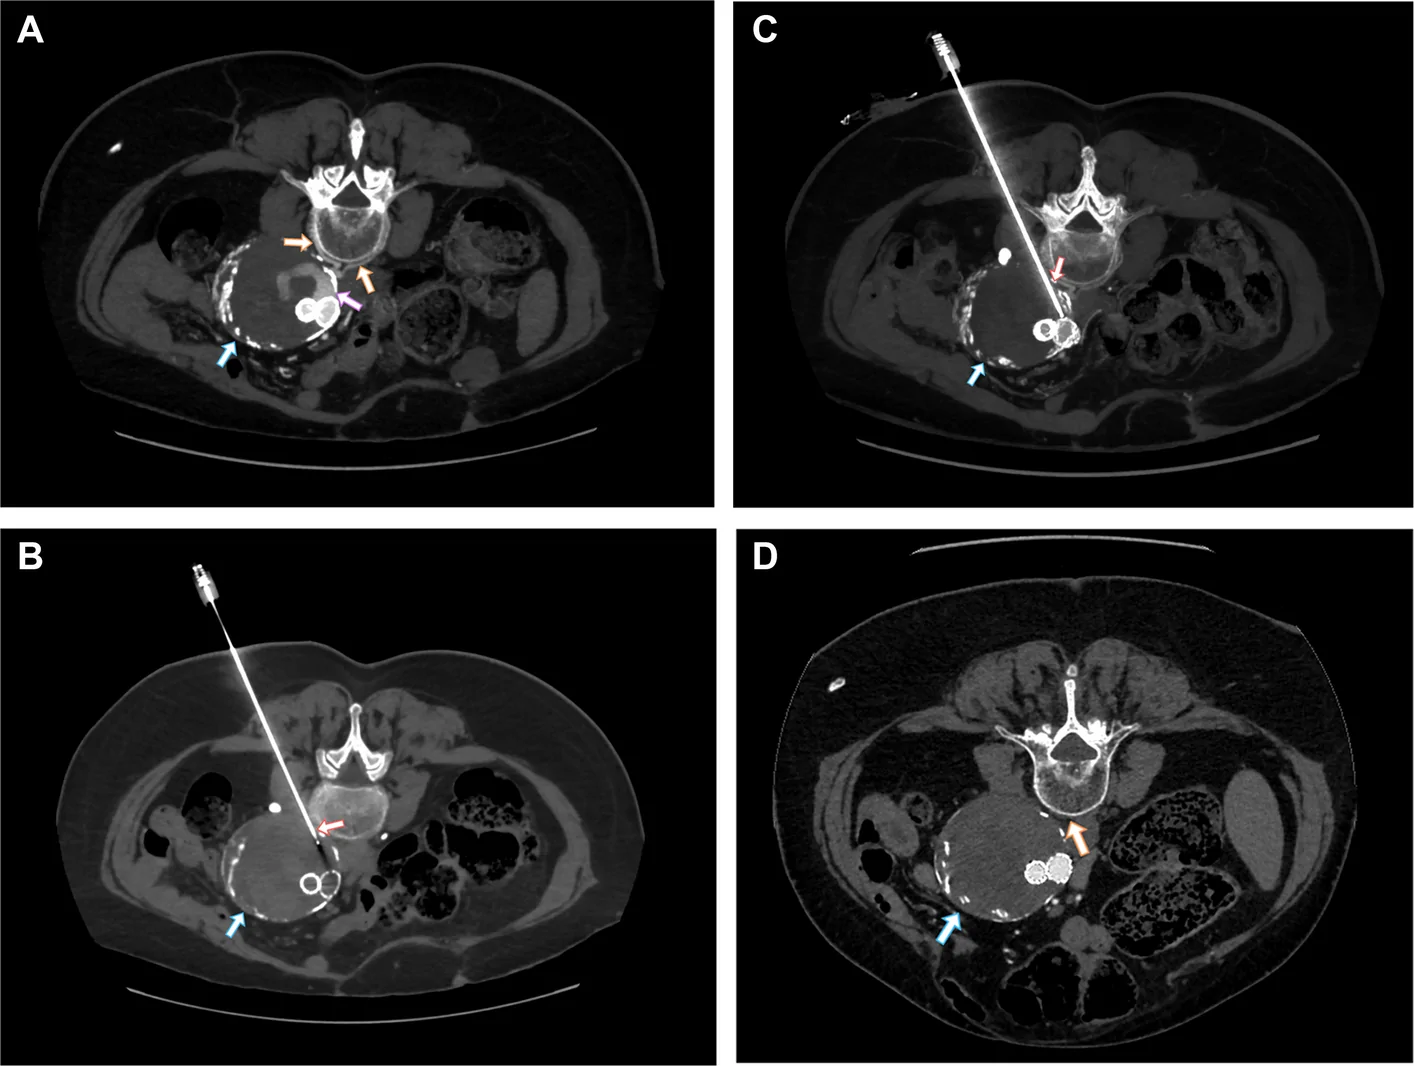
Imaging findings and procedural steps of CT-guided direct sac puncture embolization of a type II endoleak. A Contrast-enhanced CTA localizes the endoleak cavity (pink arrow) within the aneurysm sac (blue arrow) and lumbar arteries as feeding vessels (orange arrows) and facilitates planning of the percutaneous access route. B Intraprocedural CT confirms positioning of the needle tip (red arrow) within the endoleak cavity; the aneurysm sac is indicated by the blue arrow. C Immediate post-embolization contrast-enhanced CTA after injecting 4 mL of thrombin confirms the needle position (red arrow) and demonstrates no residual enhancement; the aneurysm sac is indicated by the blue arrow. D Eight-month follow-up contrast-enhanced CTA demonstrates stable aneurysm sac size (blue arrow) and no recurrent type II endoleak, orange arrow shows previous feeding vessel without contrast enhancement
